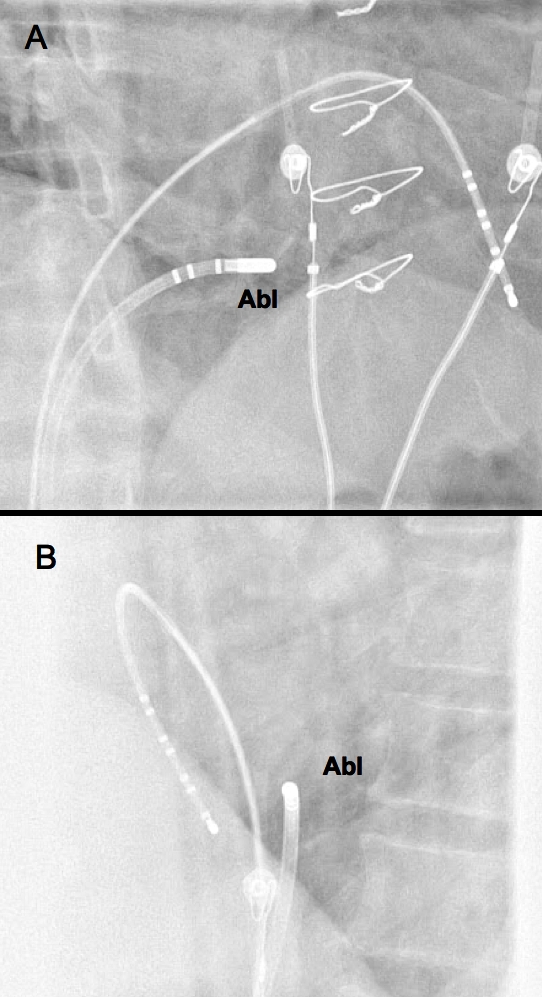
Catheter positions during successful cryoablation: ablation catheter (Abl) and right ventricular apex. Two x-ray projections are shown: right anterior oblique (A) and left anterior oblique (B)
